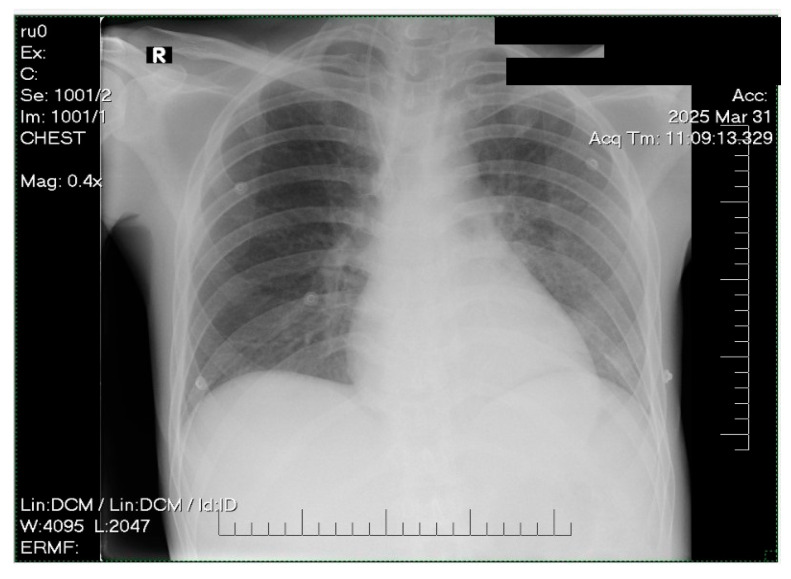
Chest X-ray showing no signs of pulmonary congestion or consolidation.
